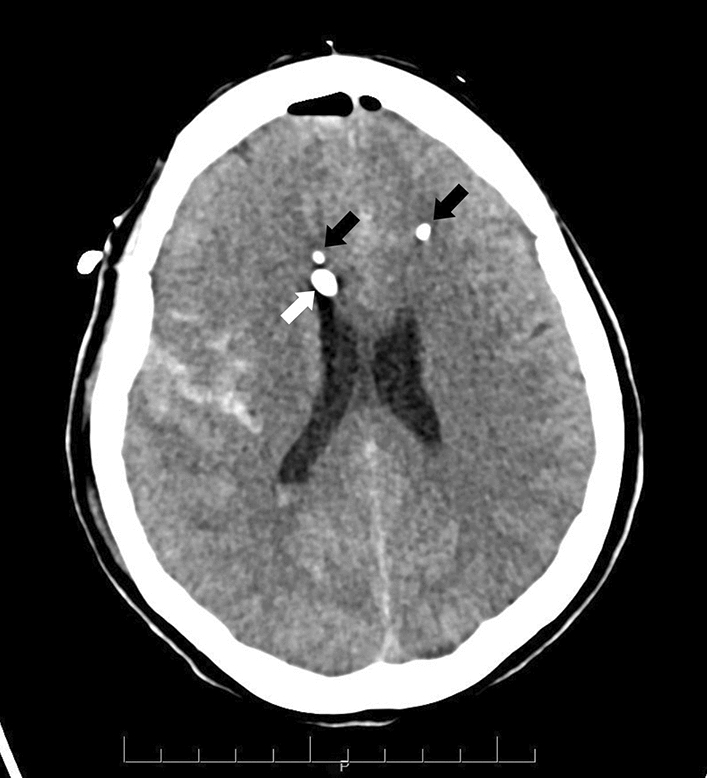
Axial cut of a cerebral CT scan of a patient with SAH. The figure shows the bilateral placement of the microdialysis probes (black arrows) and the external ventricular drain (white arrows). The CT scan has been anonymized.
